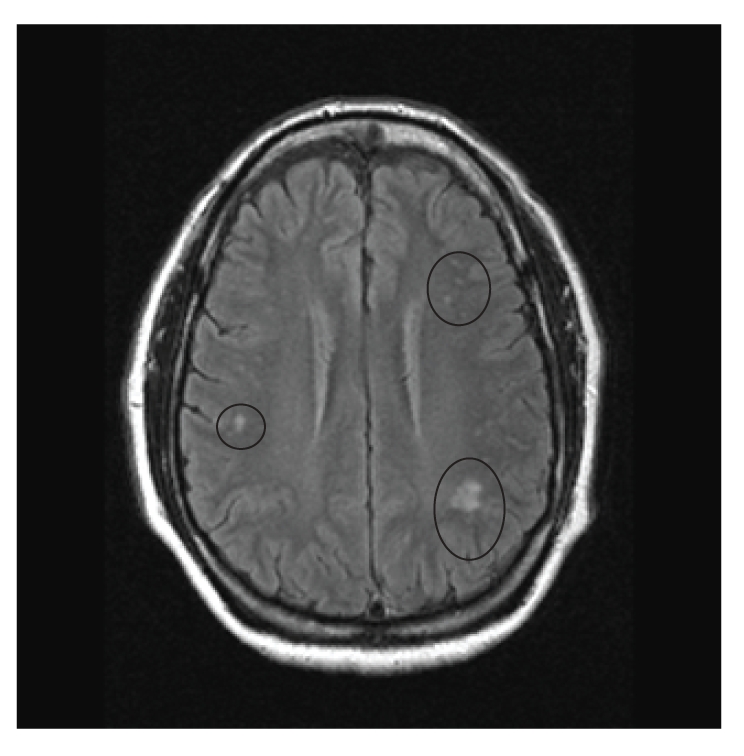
MRI image of the brain in an axial view showing the “post-contrast FLAIR image”. Note the abnormal lesions (circled) in the per ventricular area do not show any enhancement with contrast injection.
